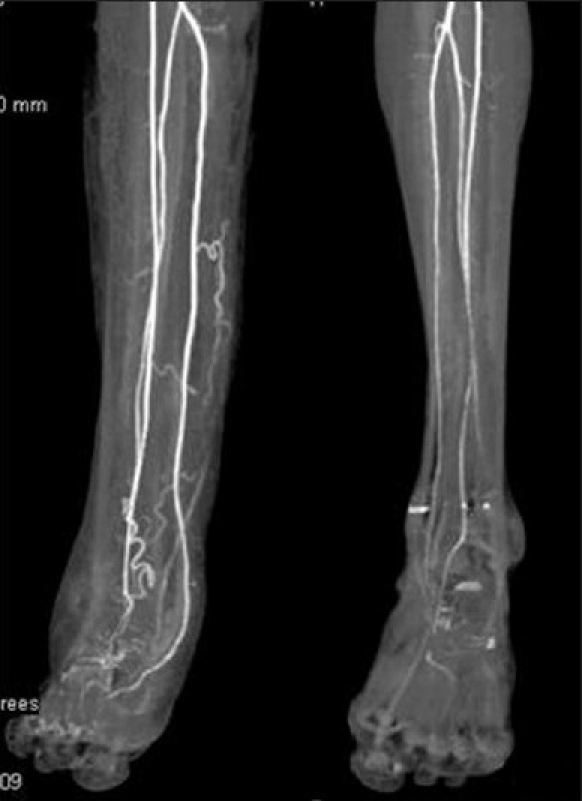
Dynamic 3D postcontrast computed tomography angiography (arterial phase) reveals hypertrophy of the right lower limb main arteries, which otherwise appear to be patent. Multiple tortuous collateral branches infiltrating soft tissues of the calf are also seen
